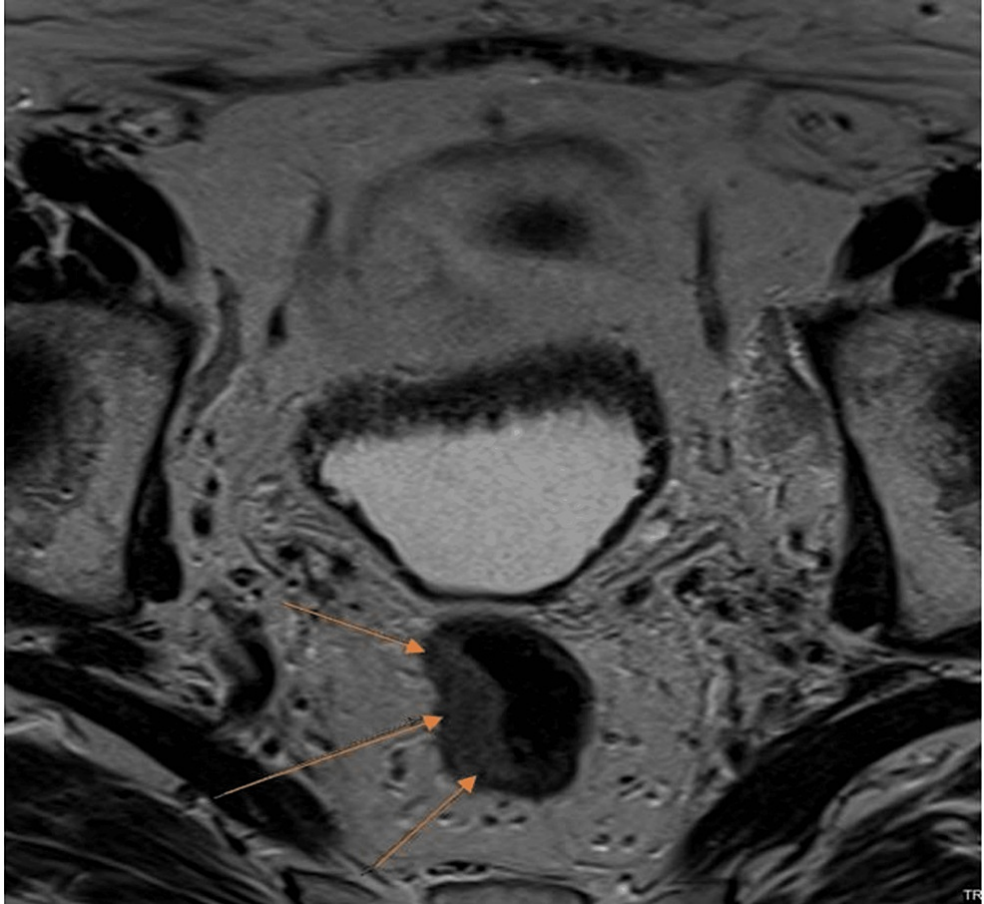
T2-weighted axial MRI image showing a rectal tumour Yellow arrows: rectal tumour, MRI: magnetic resonance imaging. Created by Collins O. Opara.
